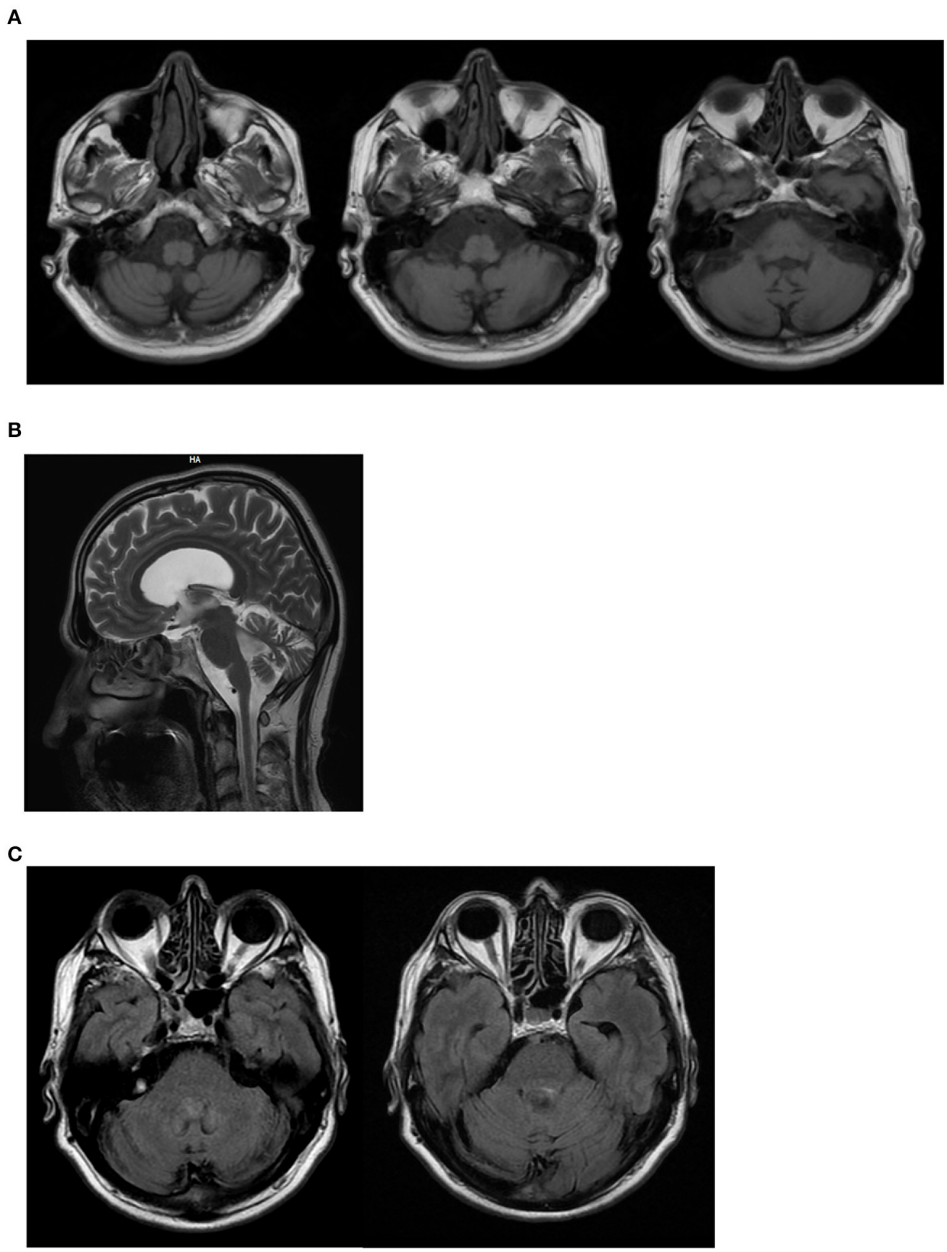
II:1 Brain MRI. (A) Axial T1-weighted shows cerebellar and brainstem atrophy; (B) Sagittal MRI scan shows global cerebellar atrophy; (C) Axial FLAIR MRI sequences shows no dentate nucleus hyperintensity.
